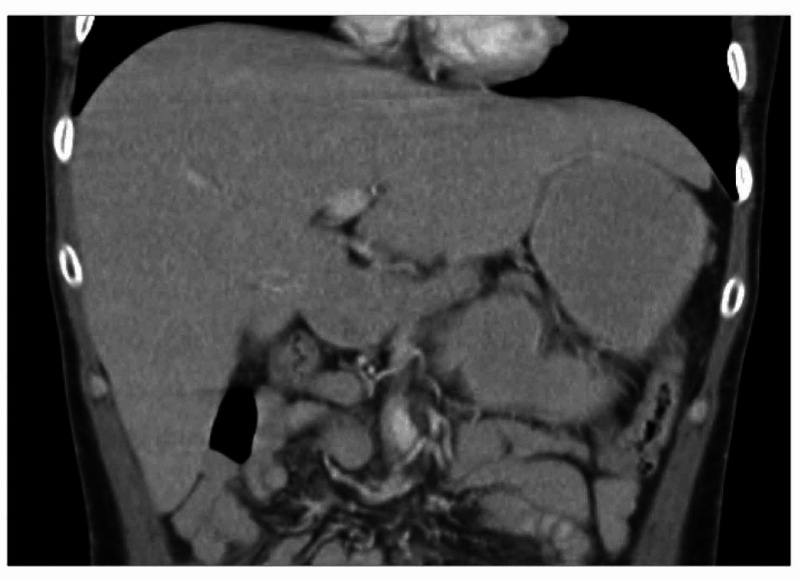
MRI of the abdomen during one of the patient's hospitalizations with improved hepatomegaly, demonstrating the reversibility of this condition
